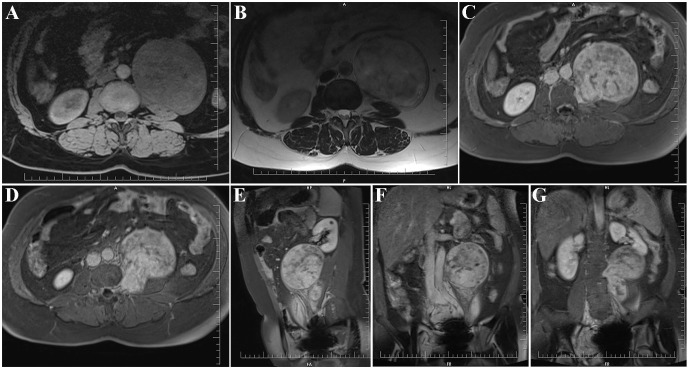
Preoperative MRI of the patient. (A) Axial T1-weighted image showing the tumor with isointense signal intensity. (B) Axial T2-weighted image demonstrating heterogeneous hyperintensity of the tumor. (C) Axial contrast-enhanced T1-weighted image revealing marked heterogeneous enhancement. (D) The tumor originates from the left L3–4 intervertebral foramen. (E) Sagittal contrast-enhanced T1-weighted image. (F) Coronal contrast-enhanced T1-weighted image showing the tumor closely abutting the abdominal aorta. (G) Coronal contrast-enhanced T1-weighted image demonstrating close contact between the tumor and the kidney and ureter, with superior displacement of the kidney.
